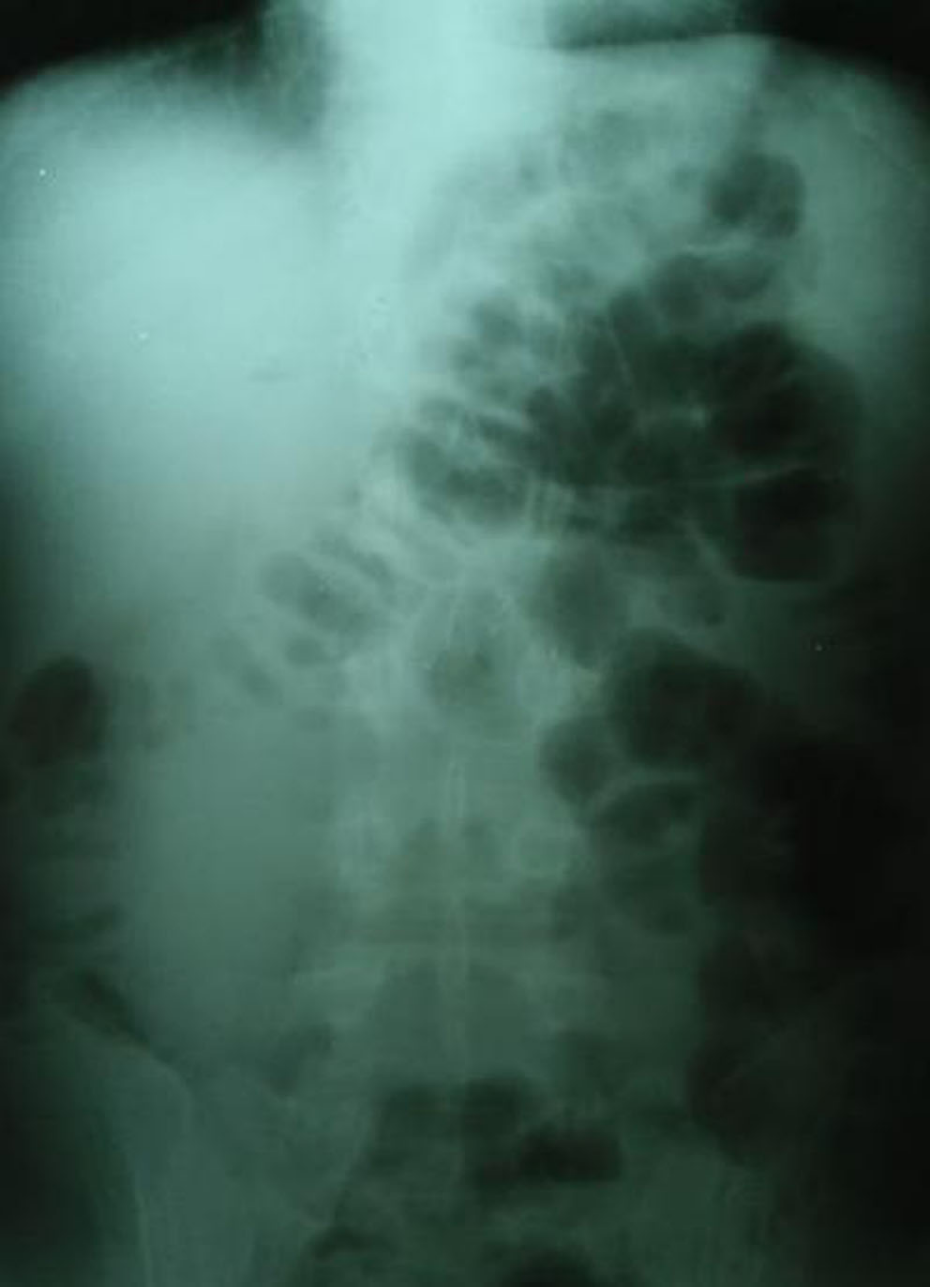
Abdominal native radiography.
